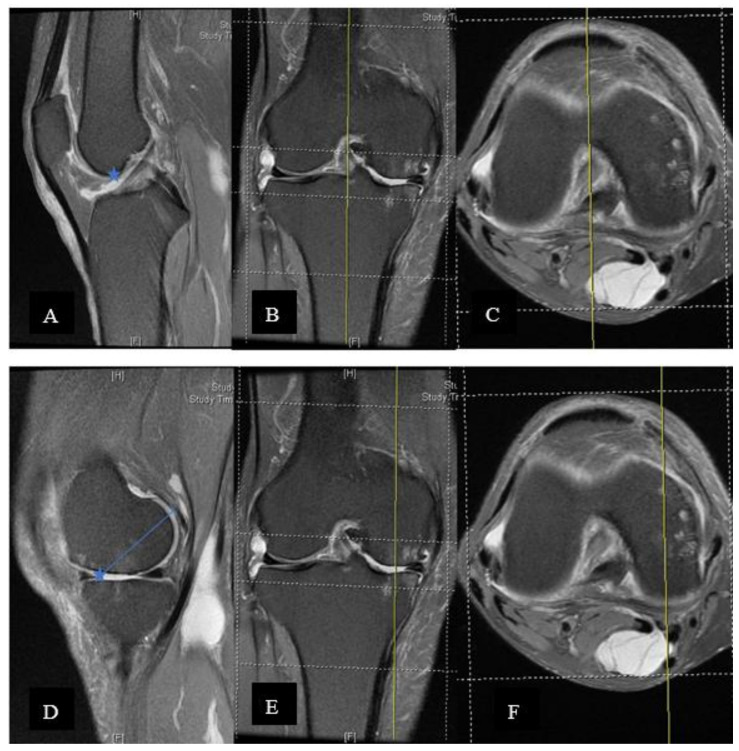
(A–C) On the sagittal section, the cut that bisected the trochlea was obtained, confirming the axial and coronal sections. The corner between trochlea and blummensant line was identified and marked (star). (A) Central sagittal cut of the knee MRI. (B) Coronal cut of the knee MRI. (C)Axial cut of the knee MRI. (D–F) The marked point was transferred to the sagittal section at the central cut of the medial femoral condyle (also have to check on the axial and coronal planes). The marked point would estimate the most anterior point of the medial femoral condyle. The most posterior point included the visualised and marked cartilage. A full thickness loss of cartilage is usually observed at the anterior region. The cartilage thickness should be added back. (D) Sagittal cut through medial femoral condyle of the knee MRI (E) Coronal cut of the knee MRI (F) Axial cut of the knee MRI.
